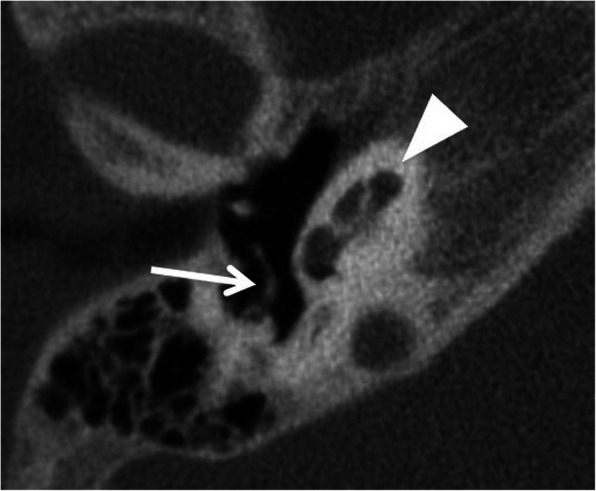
A 5-year-old male patient, with CHARGE syndrome and bilateral severe SNHL from birth. HRCT axial image shows hypoplastic cochlea type III with less than 2 turns (arrowhead). Malformed crus longum incudis and stapes are fused with the posterior tympanic wall (arrow)
